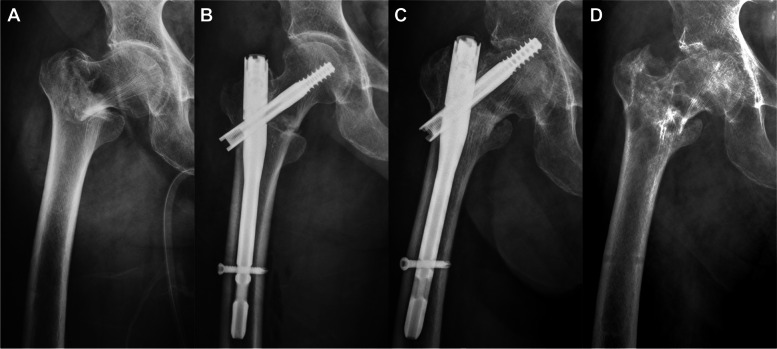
Eighty-seven-year-old female after fall down injury. A Anteroposterior X-ray image of right hip showing an extracapsular fracture (basicervical type). B The patient was surgically treated with cephalomedullary nail C But prominent cut-out was identified at postoperative 4.2 months follow-up. C Simple instrument removal was performed due to the patient’s poor general condition
